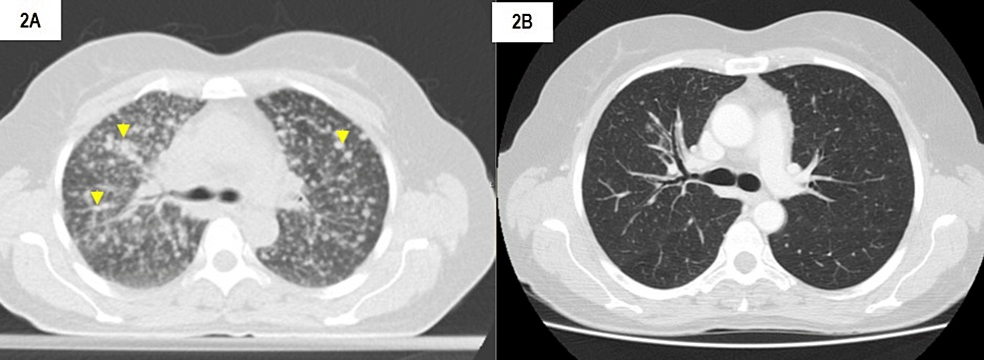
2A) Positron-emission tomography-computed tomography (PET-CT) scan image demonstrating lung metastasis at diagnosis (02/10/2017); 2B) thoracic CT scan after beginning erlotinib, showing disease response (30/11/2017); (yellow arrows: example of pulmonary lesions).
